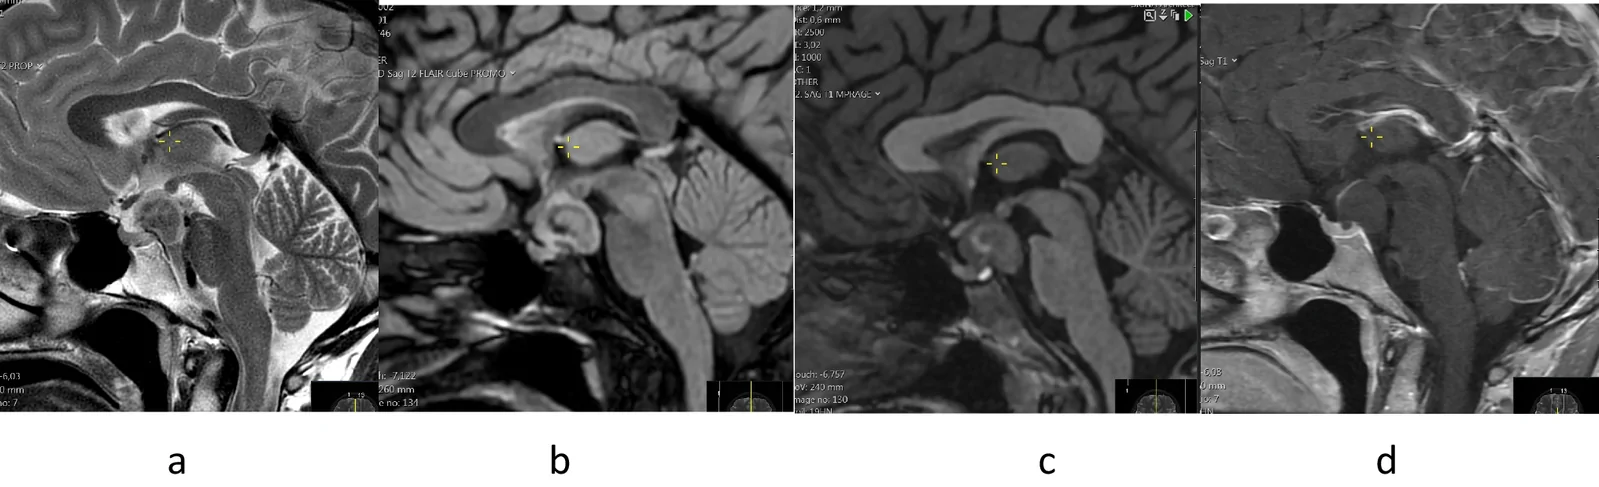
Ectopic RCC. Magnetic resonance images depict a nonenhancing suprasellar mass with an intracystic nodule that displays hypointensity on sagittal Flair and T2-weighted images (a, b) and iso- to slight hyperintensity on the sagittal T1-weighted image (c). There is no enhancement on the sagittal T1-weighted image after contrast administration (d). The lesion is located just dorsal to the pituitary stalk, with a small extension into the pituitary gland, and occupies the preopontine cistern
